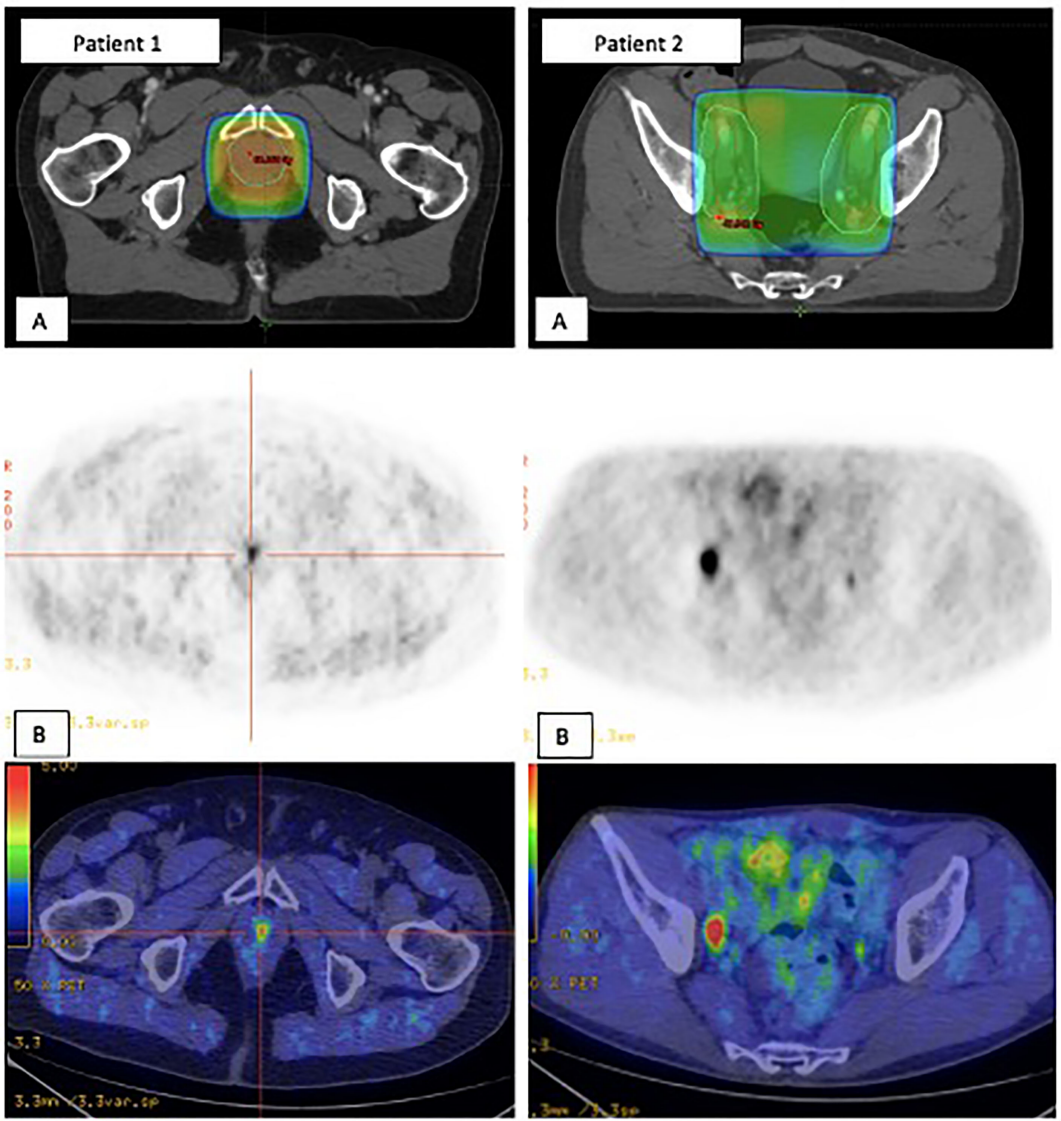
Two patients’ examples of in-field recurrences (A) which were positive on Choline PET/CT (B). Patient 1. Urethral anastomosis failure within the PB RT volume. Patient 2. Right iliac extern nodal failure within PLN RT volume. PB, Prostate bed; PET-CT, Positron emission tomography-computed tomography; PLN, Pelvic lymph nodes; RT, Radiotherapy.
